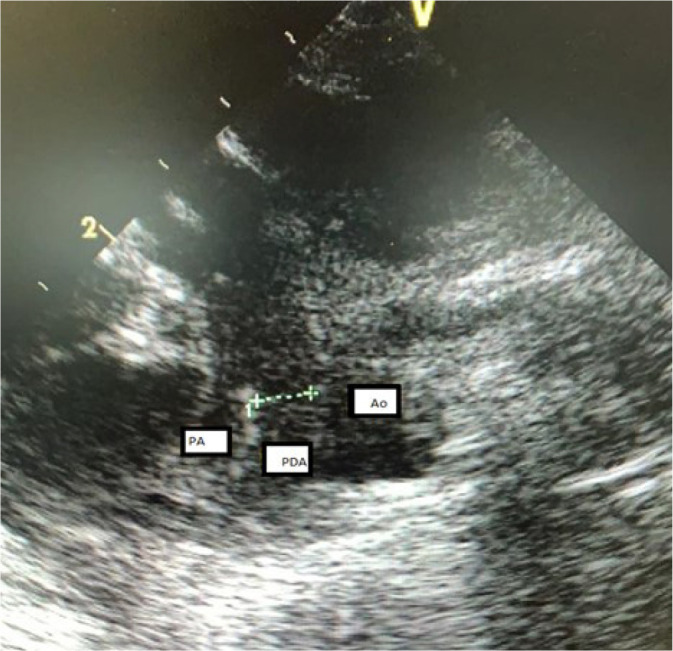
Transthoracic echocardiogram. Ao=aorta; PA=pulmonary artery; PDA=patent
